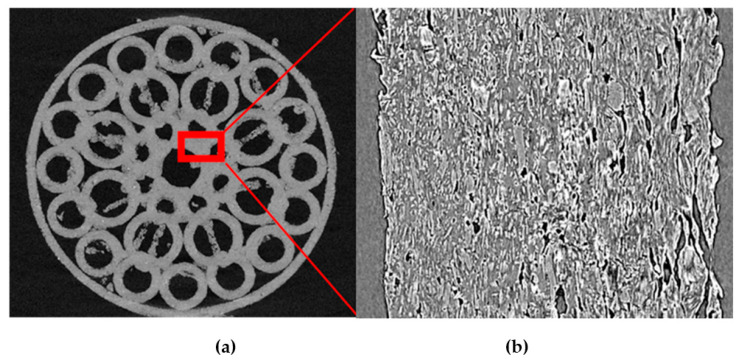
X-ray tomography. (a) Image of the 3D printed structure. (b) Detailed of one toroid of the structure.
